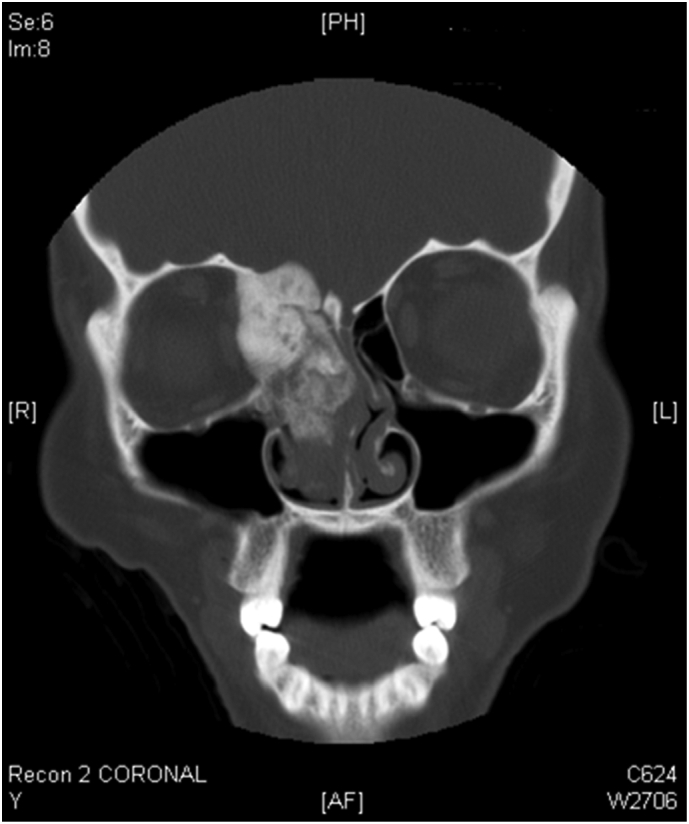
Paranasal coronal CT scan shows right ethmoid sinus and nasal cavity mass with orbital and intracranial involvement.
